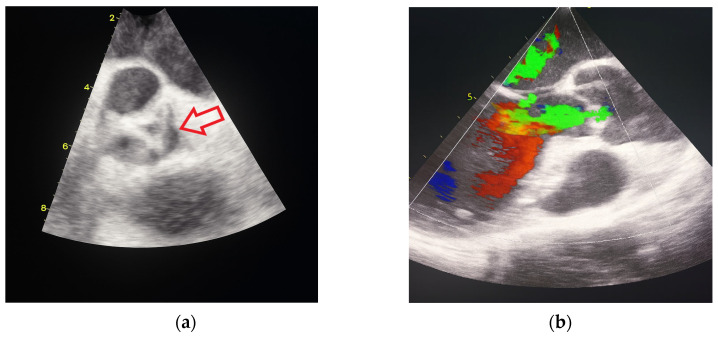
Transesophageal heart ultrasound 2D imaging: (a) degeneratively changed bicuspid aortic valve with rudimentary left coronary cusp (red arrow). (b) 2D visualization of moderate aortic regurgitation in the context of a pathologically altered aortic valve.
